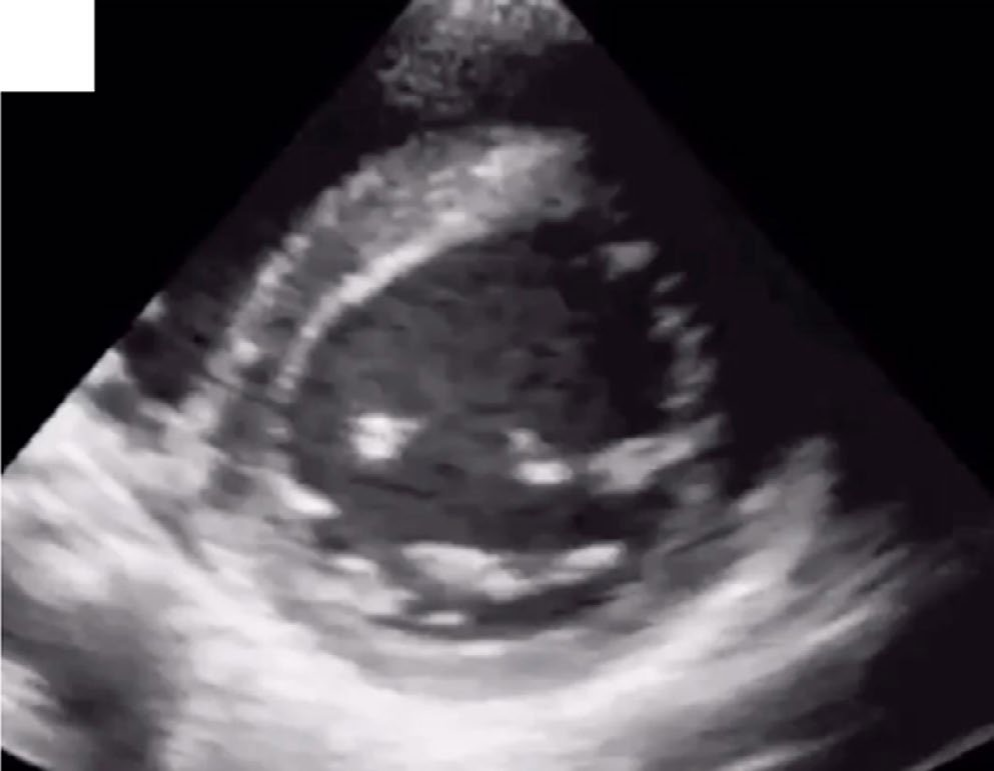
Echocardiography showed hypokinesia in anterior circulation with severe reduction in left ventricular systolic function
